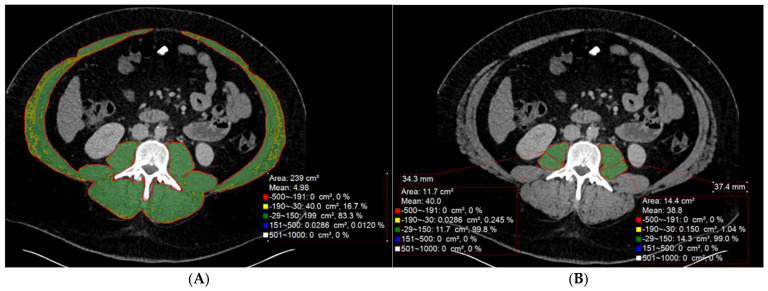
CT-Based Body Composition Assessment at the L3 Level. (A): The image demonstrates CT-based segmentation and assessment of the abdominal skeletal muscle area at the L3 level. (B): The image demonstrates CT-based segmentation and assessment of the psoas muscle areas, attenuations, and thicknesses.
